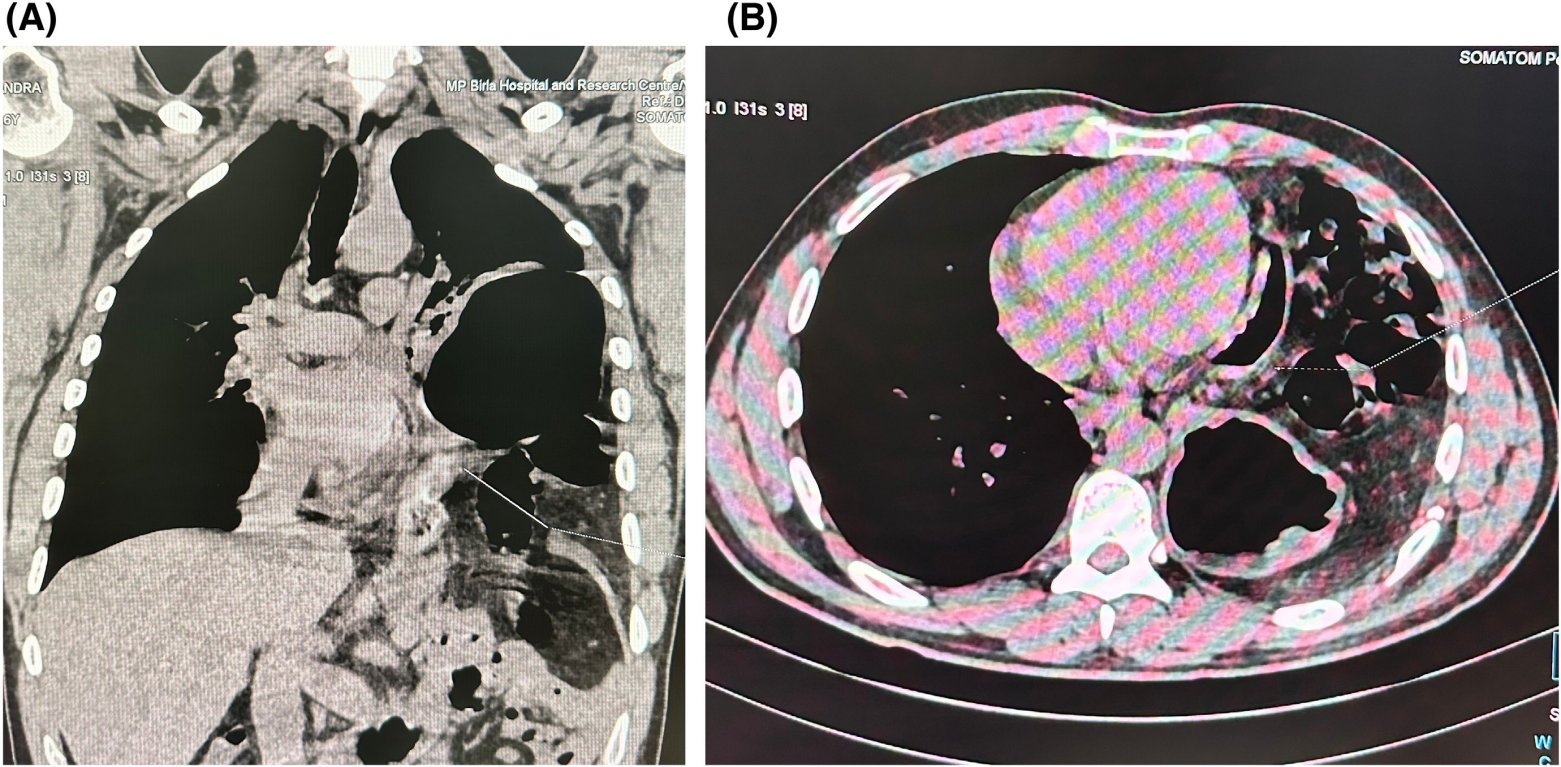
CT chest (A) coronal view and (B) axial view‐ showing left diaphragmatic rupture and herniation of stomach, small bowel, and spleen into left hemithorax.
